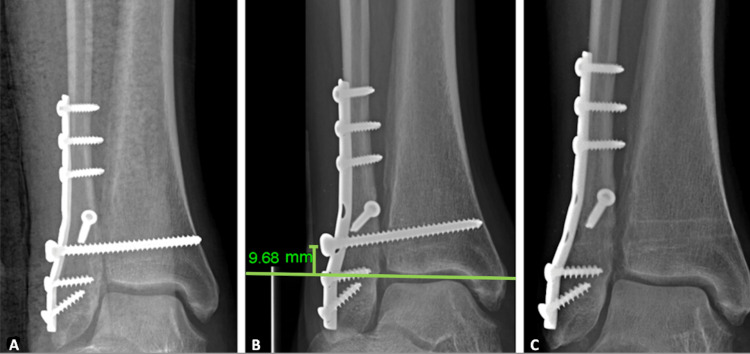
Full radiograph sequence All radiographs are from a mortise view. Images of a 53-year-old male with a BMI of 66 are shown. Postoperative radiographs using a fibular plate and a single 4.5 mm syndesmotic screw are shown (A). A radiograph demonstrating the measurement of the height of screw placement of 9.68 mm above the tibiotalar joint (B). The syndesmotic screw was removed 15 weeks later due to persistent pain and discomfort (C).
